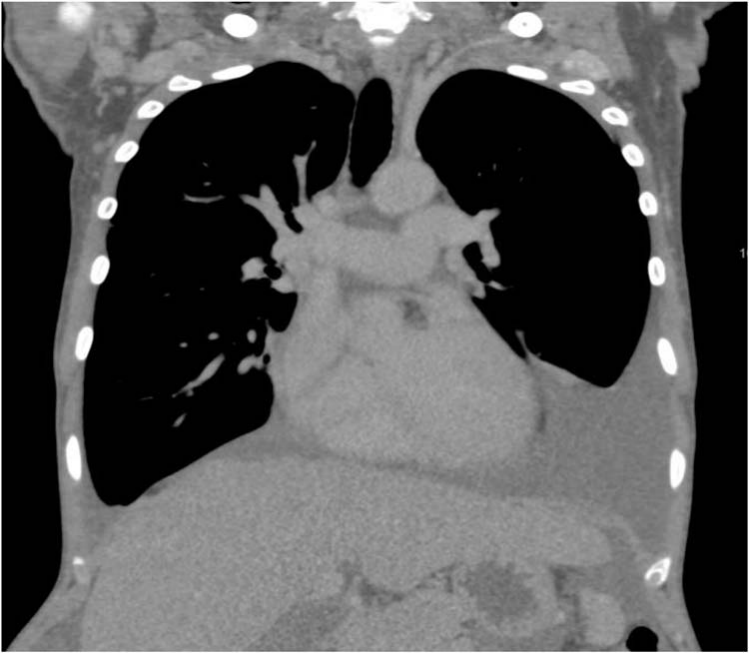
CT scan of the chest shows pleural effusion.
